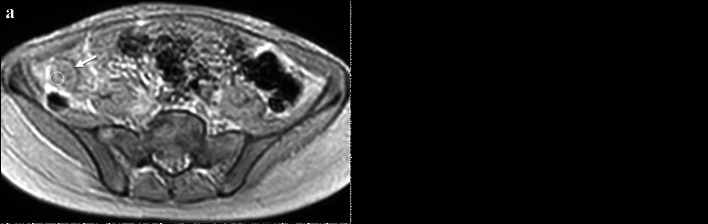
A 12-year-old female with Crohn’s disease. a Axial magnetization transfer (MT) MR image without an off-resonance radiofrequency (RF) pulse shows that the ileal wall is hyperintense (*arrow*). b Axial MR image with an off-resonance RF pulse shows loss of signal intensity in the bowel wall (*arrow*), presumably due to the presence of macromolecules. Regions of interest (white circles) were placed in the bowel wall on both sequences to calculate the MT ratio (45% for this patient)
